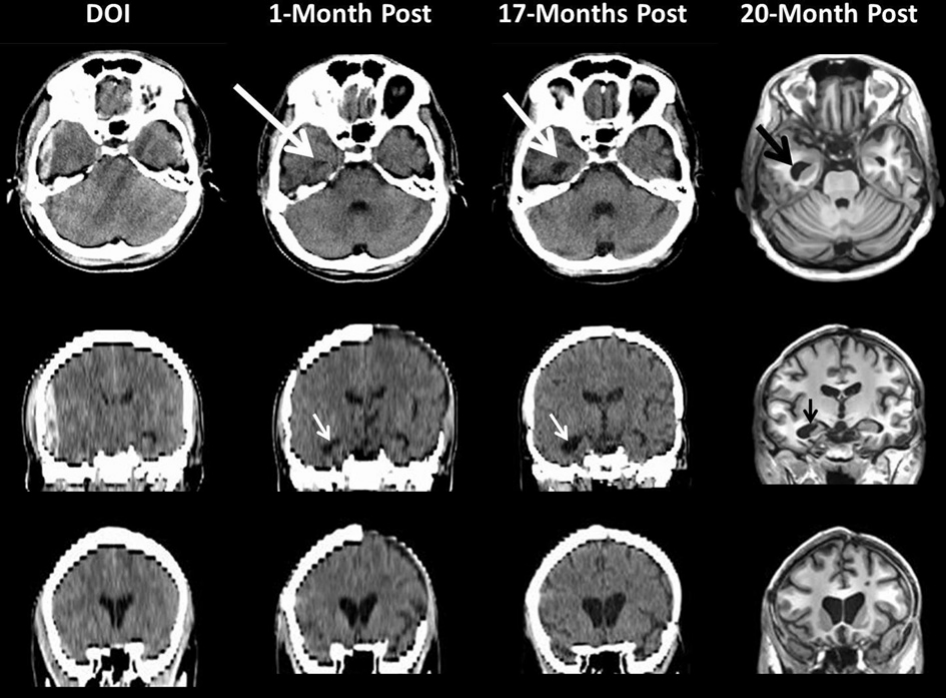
This patient sustained a severe TBI as a consequence of a fall. Note on the day of injury (DOI) the computed tomography (CT) scan demonstrates the presence of a large epidural hematoma with brain displacement. Repeat scanning was performed at 1-month (CT scan), 17 (CT scan) and 20 months (MRI) post-injury. For the CT scans in the middle and bottom rows the coronal sections shown are based on re-sampled axial images with degradation in image resolution but sufficient to depict ventricular changes over time. Note how in the DOI scan the temporal horn is basically undetectable from parenchymal shift from the epidural as well as edema but clearly visible and dilated by 1-Month (white arrow) which increases by 17-months and even more prominent by 20 months as shown in the MRI findings. The bottom coronal images clearly depict increasing dilation of the anterior horns of the lateral ventricular system reflecting brain parenchymal volume loss that progresses from DOI through 20-months post-injury. Note at 1 month the patient still has missing bone-flap from the original craniotomy to treat a contra coup hemorrhagic contusion and subdural hematoma.
